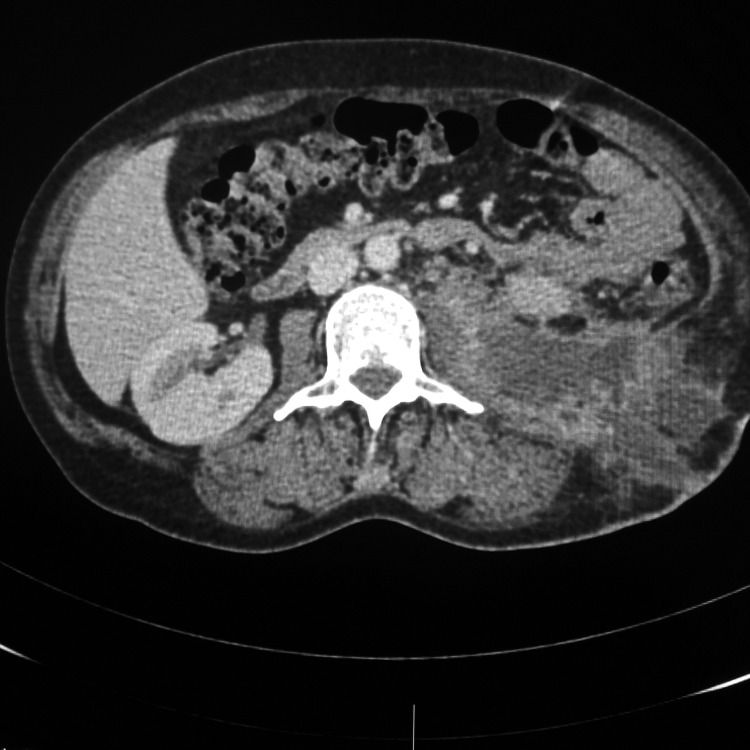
Sagittal section of CT of the abdomen showing large retroperitoneal and subcutaneous pus collection arising from the left kidney.
